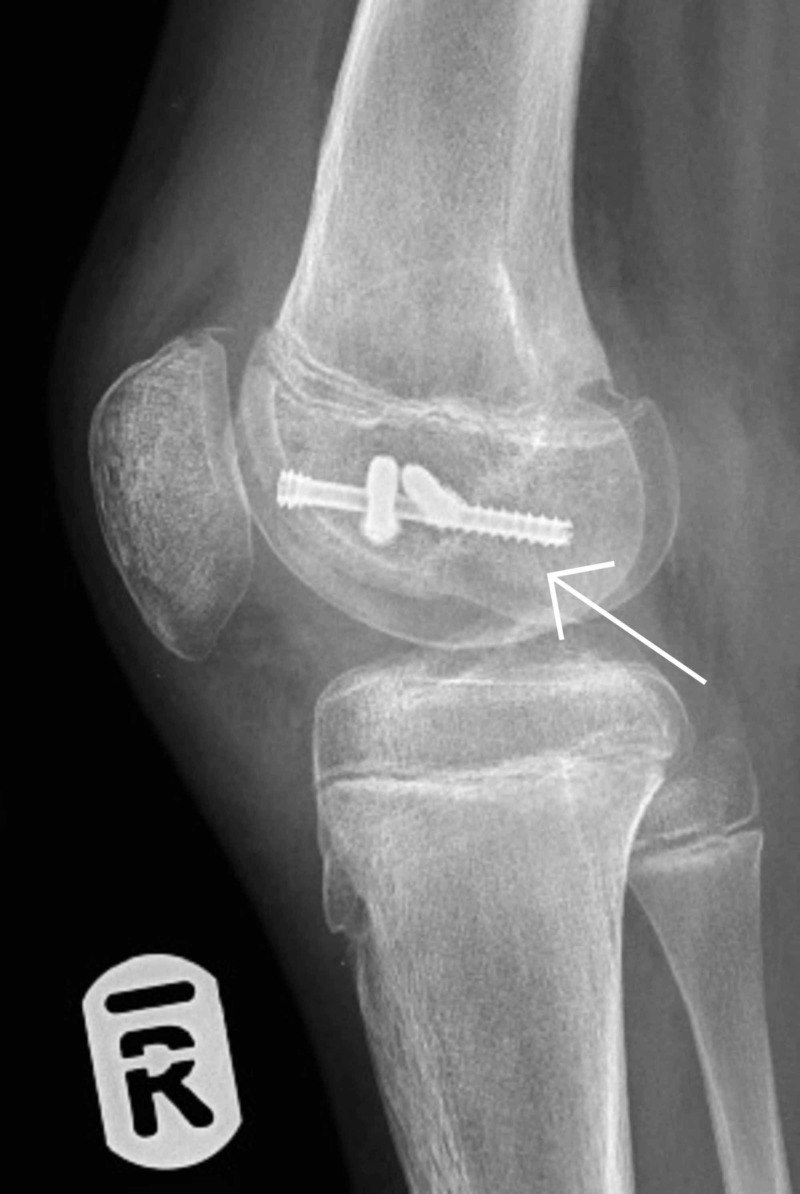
Post-operative lateral x-ray post Kirschner wire removal
